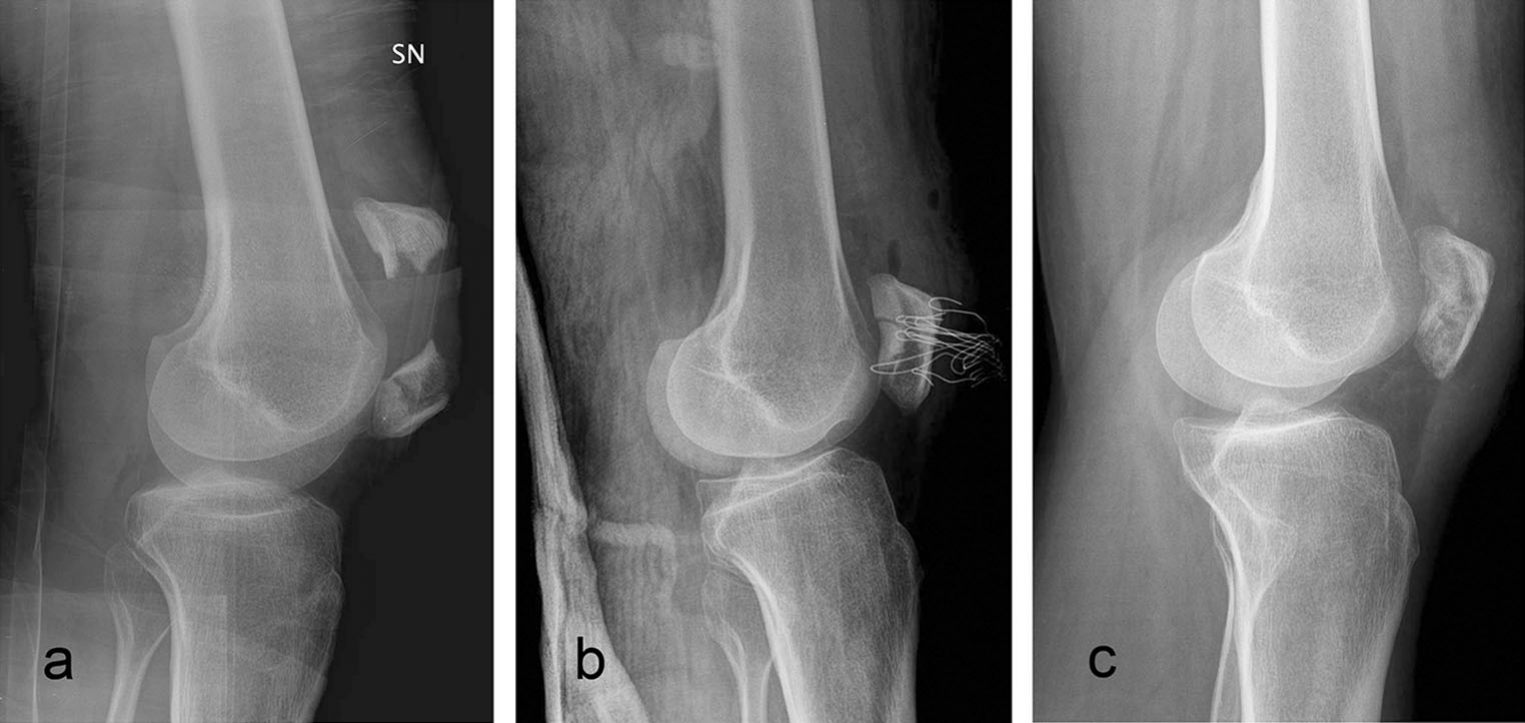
Transverse patellar fracture. Preoperative X-ray (a); postoperative X-ray (b); final follow-up X-ray (c)
